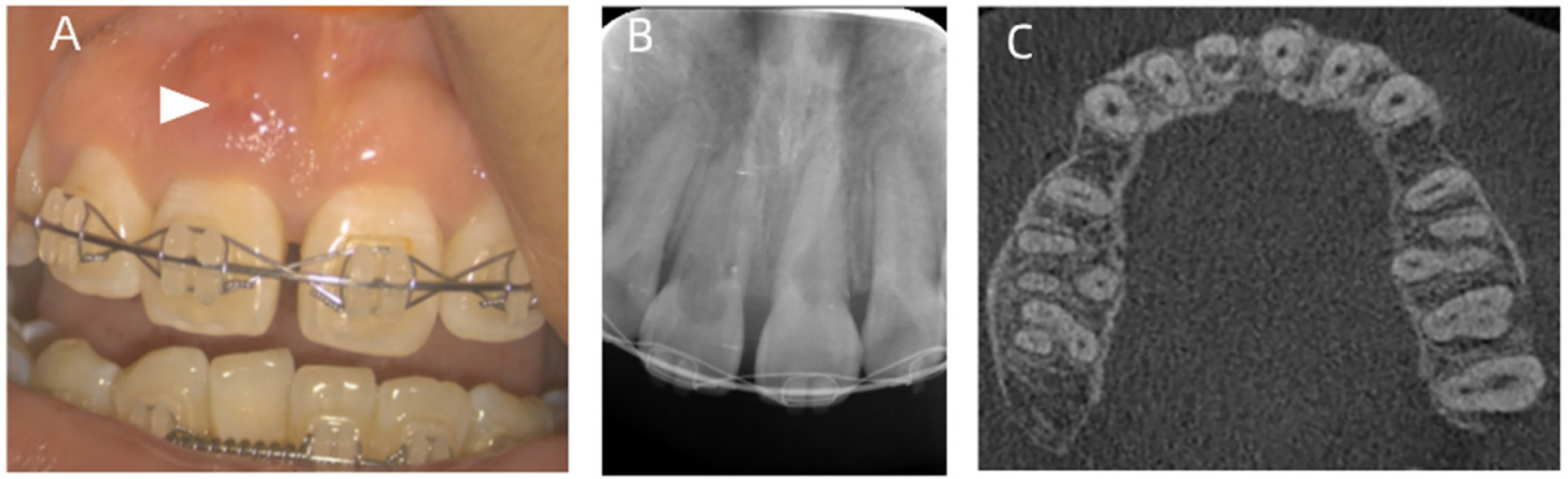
Oral examinations performed before root canal treatment. (A) Intro-oral photographs before root canal treatment. The white arrow points to the sinus on buccal mucosa. (B) An x-ray shows that in the region of the pulp chamber to the apical quarter of the root, an irregular low-density image area is observed in the upper right central incisor. In the root canal of the upper left central incisor, a semicircular low-density area is observed. (C) A CBCT examination of the initial dental status. The pulp chamber and the root canal of the upper right central incisor are absorbed, the walls of the root canal are thin, and the thickness is about 1 mm.
